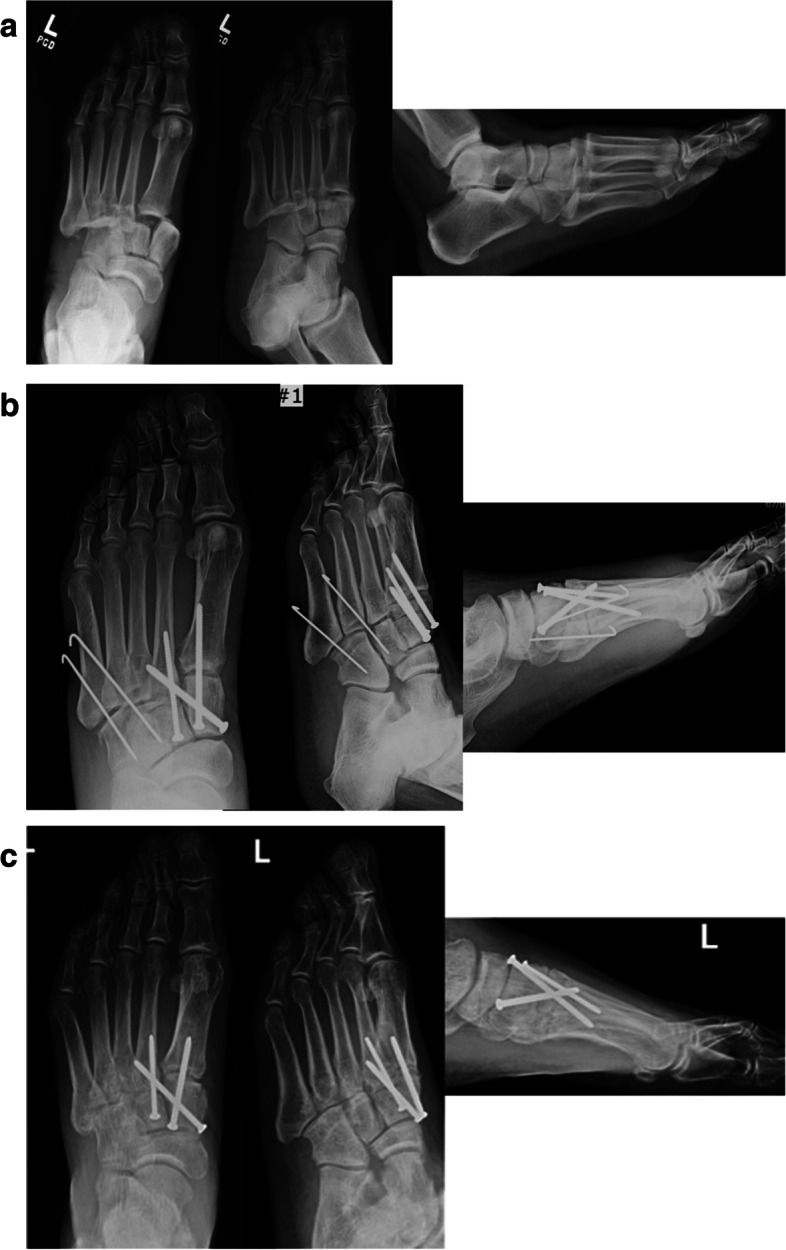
a Anteroposterior, oblique, and lateral injury radiographs of left foot demonstrating Myerson type A laterally incongruous TMT joint fracture-dislocation. b Anteroposterior, oblique, and lateral postoperative radiographs demonstrating restoration of anatomic alignment of midfoot, medial column TMT joint primary arthrodesis and lateral column K-wire fixation. c Anteroposterior, oblique, and lateral postoperative radiographs demonstrating removal of lateral column K-wires and mild narrowing of fourth and fifth TMT joints
